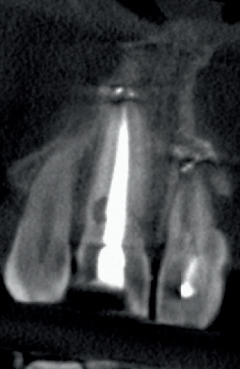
The CBCT allows for a more precise delineation of the lesion's extent at 17 years.
